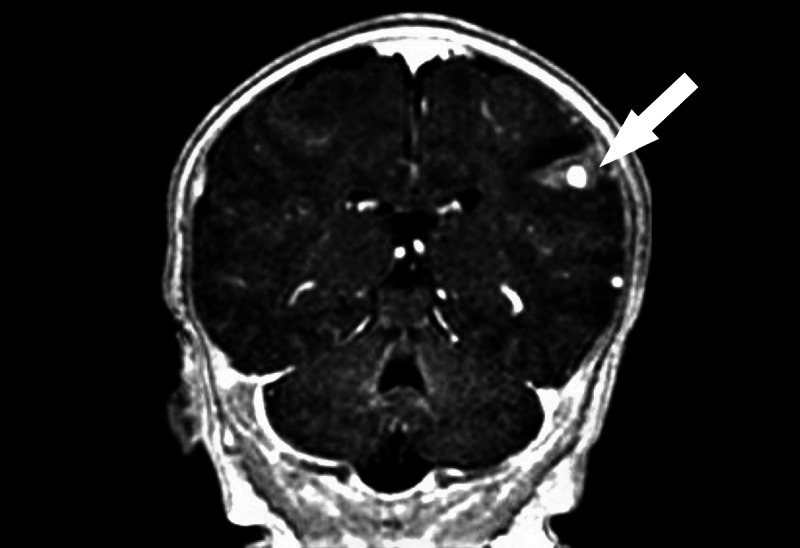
Pre-embolization MRI of the brain MRI of the brain depicting a dysplastic superficial intra-axial aneurysm arising at the distal left middle cerebral artery (MCA) branch with hemorrhage of the left posterior frontal lobe anterior to the central sulcus (white arrow)
